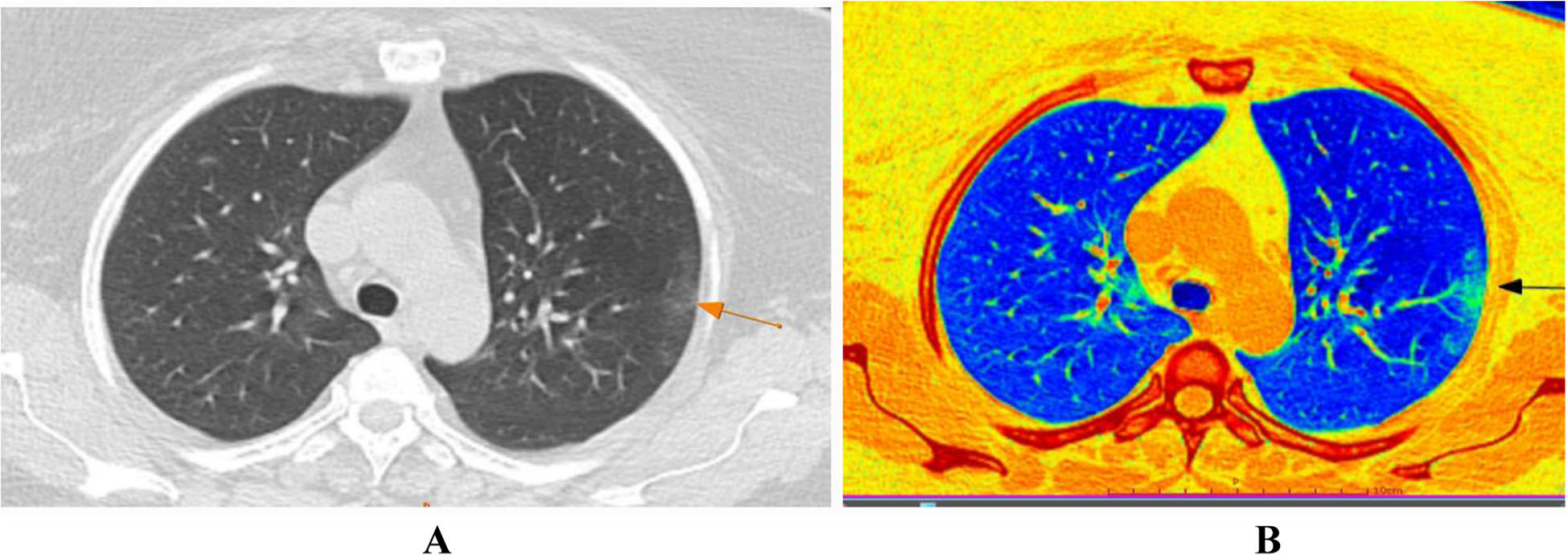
A 40-year-old female patient with first presentation with minimal cough and sore throat; CT-SSS was 2. Follow-up revealed complete resolution of the symptoms after 13 days from the onset. a MDCT chest, axial cuts show suspected faint peripheral sub-pleural patchy ground glass opacity seen in the left lung upper lobe apico-posterior segment; confirmed by the color coded images (b) with HU more than − 750
